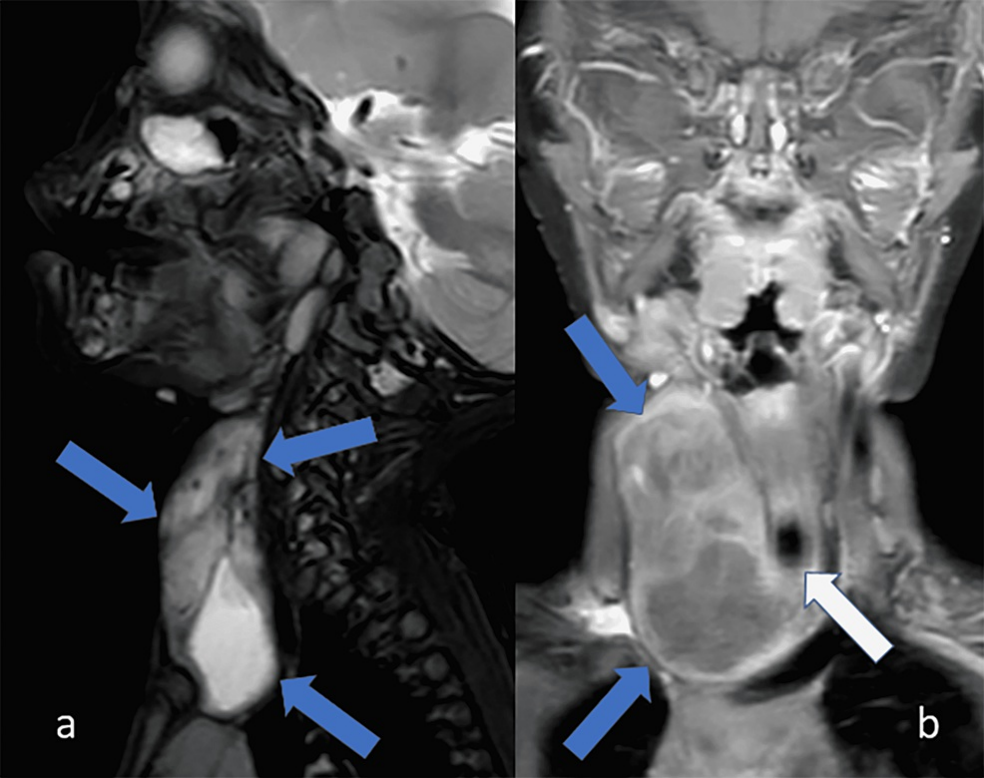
(a) Sagittal T2-weighted image with fat saturation demonstrates the large, heterogeneous lesion extending from the mid cervical region to the thoracic inlet (blue arrows). (b) Coronal post-contrast, T1-weighted image with fat saturation demonstrates the partially enhancing, cystic-appearing lesion in the thyroid gland (blue arrows) displacing the trachea to the left (white arrow). Image courtesy: This image was provided by Dr. Laura L. Hayes from the radiology department at Nemours Children’s Health System.
